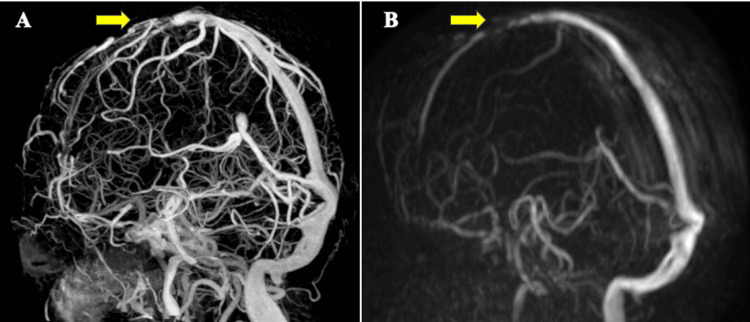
Computed tomography venography (CTV) of the head on admission and magnetic resonance venography performed 11 days later. A. CTV revealed a contrast defect in the superior sagittal sinus; B. Magnetic resonance venography performed 11 days later demonstrated slightly improved visualization of the superior sagittal sinus compared to the initial CTV.
